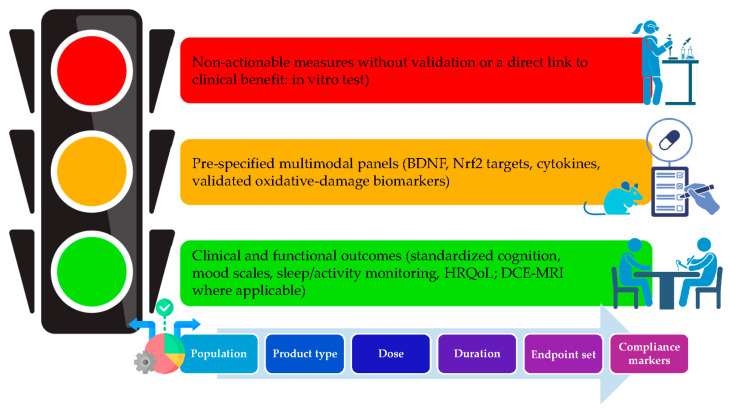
From mechanism to claims—endpoint hierarchy and trial-design decision tree. BDNF: Brain-Derived Neurotrophic Factor; Nrf2: Nuclear factor erythroid 2; HRQoL: Health-Related Quality of Life; DCE-MRI: Dynamic Contrast-Enhanced Magnetic Resonance Imaging. Figure created with assistance from BioRender (https://biorender.com/; accessed on 14 September 2025), image concept, improvements and curation performed by the author.
